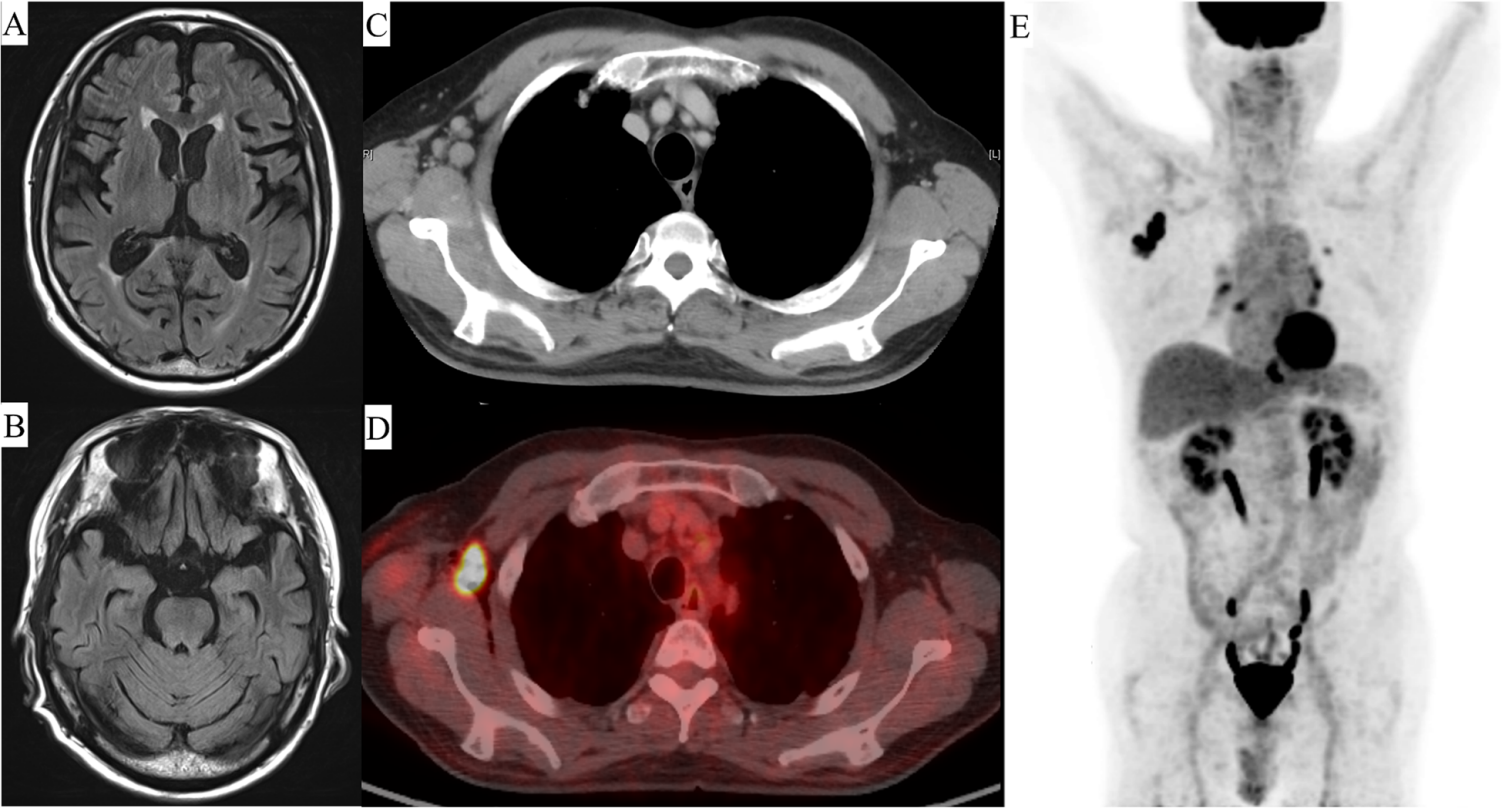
Image findings. a Magnetic resonance imaging on admission to hospital reveals no parenchymal signal change. b Computed tomography (CT) before treatment reveals an enlarged lymph node in the right axilla (1.9 × 2.2 mm). c
